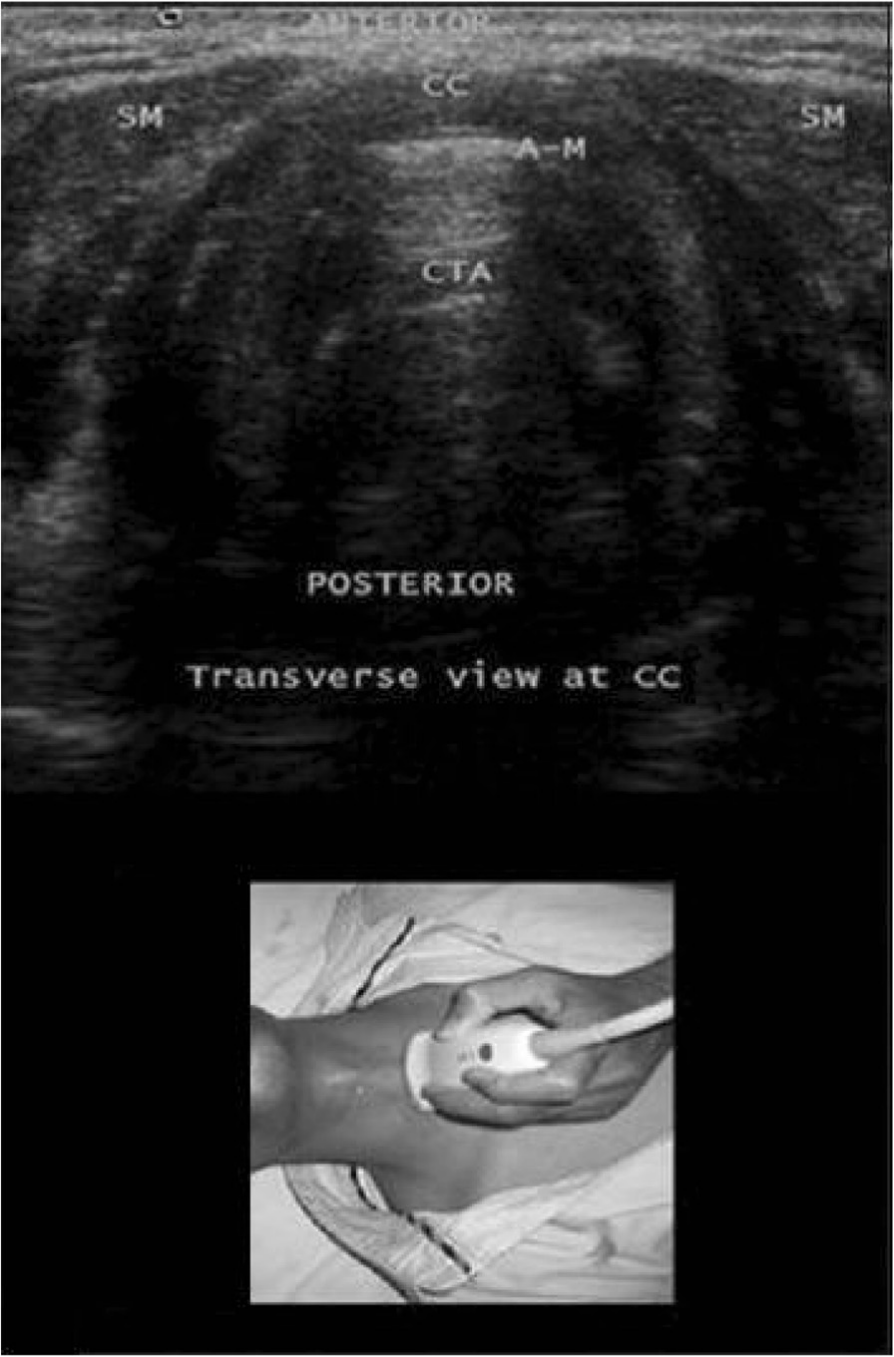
Transverse view at the cricoid cartilage. A-M, air mucosal interface; CC, cricoid cartilage; CTA, comet tail artefacts; SM, strap muscles. Reprinted with permission from Wolters Kluwer [42]
